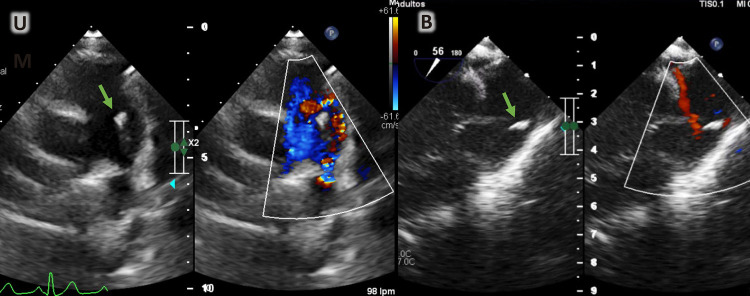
A) ETT dia 30: podemos observar shunt de PCA (cor vermelha) e vegetação no TP (seta verde). B) ETT. No 40º dia: visualização do fluxo de entrada e saída do ventrículo direito do esôfago médio; Doppler 2D e colorido mostram massa hiperecoica compatível com vegetação (seta verde) no TP.
